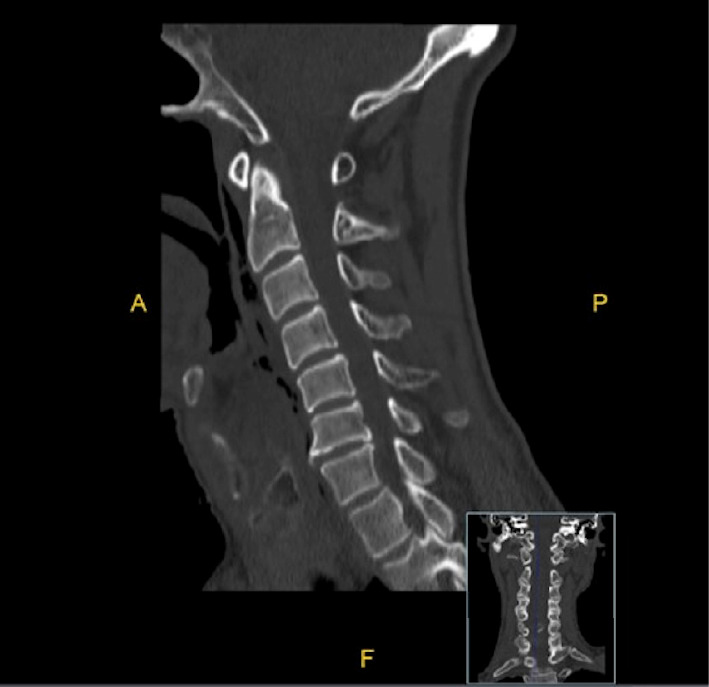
Sagittal CT scan of the soft tissue of the neck showing a longitudinal tear in the posterior esophagus from the beginning of the hypopharynx to the C5 vertebra.
